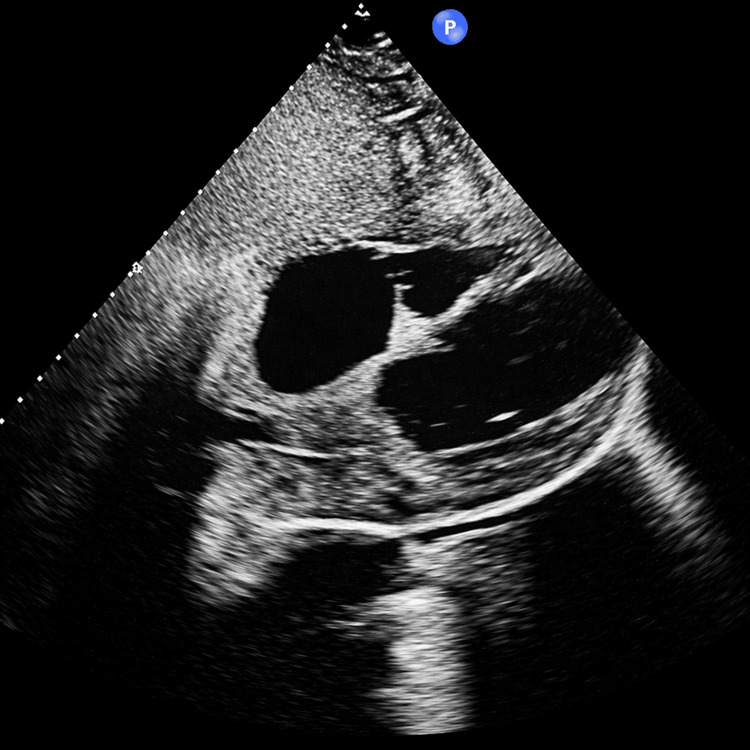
The follow-up echocardiography Transthoracic echocardiography demonstrated normal cardiac function with no pericardial effusion.
